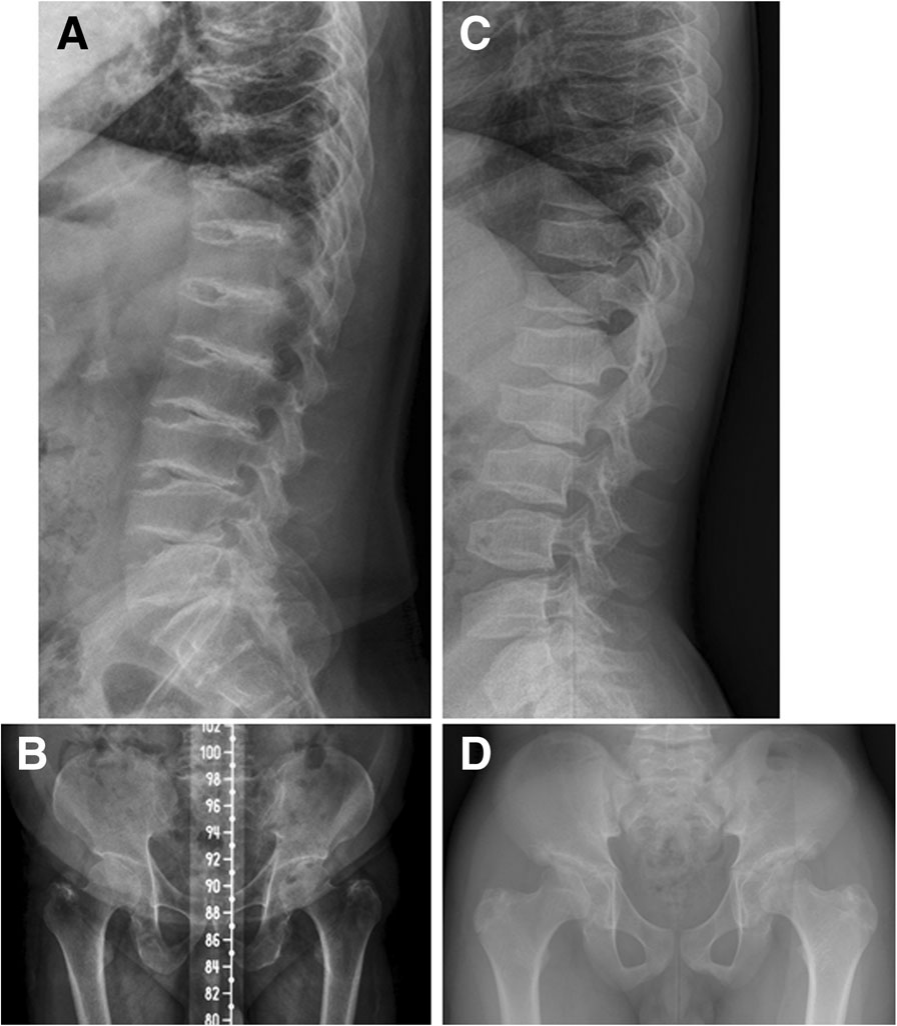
Lateral spine and hip radiographs of two SEDT-XL patients. a, b Radiographs taken from the proband with the c.1A > T, *TRAPPC2* variant at age of 45 years. a Thoracolumbar lateral spine shows uniform platyspondyly and hump-like bony projections at the centroposterior portions of upper and lower endplates, and narrowing or almost obliteration of the intervertebral disc spaces. b Pelvis radiograph shows narrow iliac wings with relatively long ischium and pubic bones, deep acetabulum and degenerative osteoarthtis with narrow hip joints. c, d Radiographs from the proband with the c.40delG, *TRAPPC2* variant at age 15 years. c Spine lateral radiograph shows platyspondyly with elongated vertebral bodies and hump-like bony protrusion at the upper and lower endplates, dominantly noted in the lumbar spine. d Hip shows relatively long ischium and pubic bones. Deep acetabulum and dysplastic femoral heads with premature degenerative hip joint narrowing are noted
